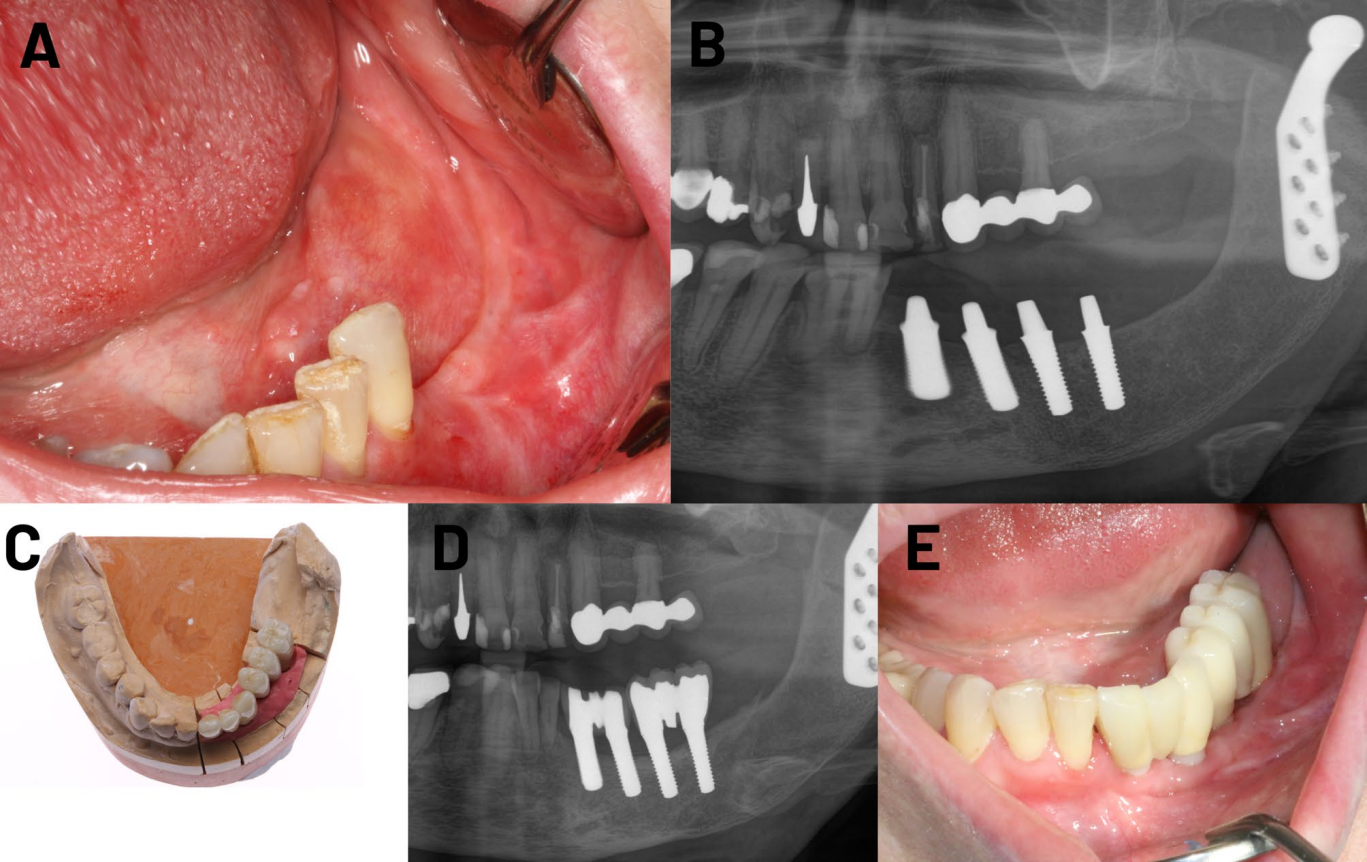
(A) This patient underwent a lower-jaw resection of the lift mandible due to osteomyelitis. The clinical picture shows the missing teeth and the reconstruction in the lower jaw using vascularized fibula flap. (B) The X-ray shows the lower jaw after implant placement. The patient was treated with four zirconia implants. (C and D) After a 3-month healing period, the implants were loaded onto a fixed dental bridge. (E) Clinical picture after one-year follow-up with the final dental crowns.
